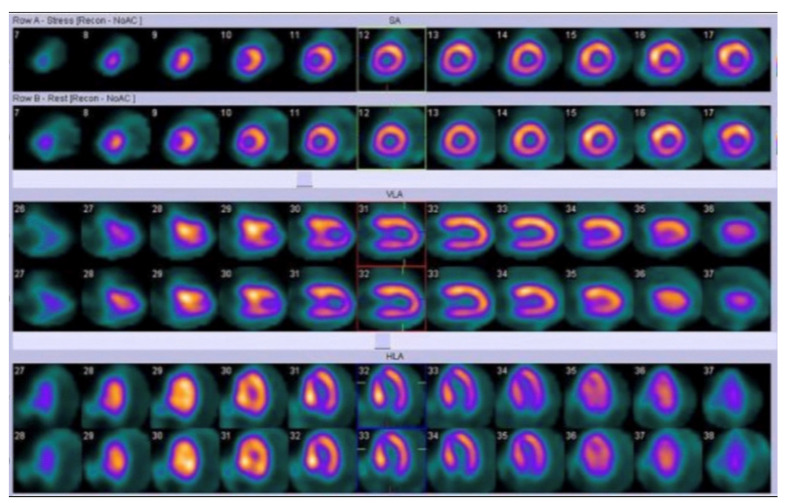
Myocardial perfusion imaging with Single Photon Emission Computed Tomography (SPECT) in a pediatric patient: both stress and rest images (axial images in first and second lines, vertical long axis images in third and fourth lines, horizontal long axis images in fifth and sixth lines) show left ventricular posterior wall and septal hypertrophy [98].
